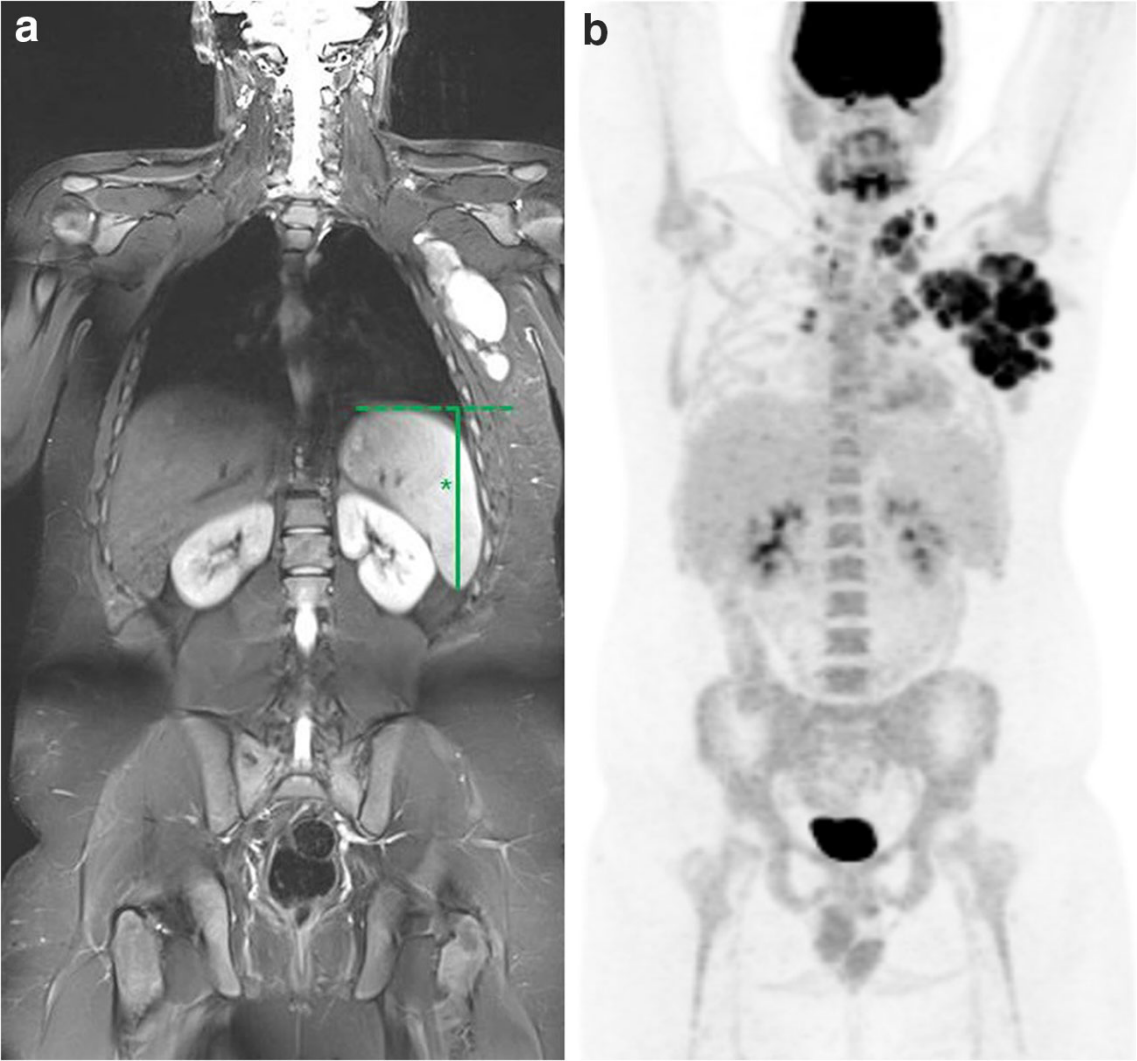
A 17-year-old boy with stage II Hodgkin lymphoma. Example of upstaging by WB-MRI due to enlargement of the spleen without focal lesions. Coronal T2-weighted WB-MRI (a) shows enlargement of the spleen without focal lesions (*size = 14.1 cm). Coronal maximum intensity projection (MIP) of the FDG-PET (b) demonstrates no involvement of the spleen. The involved lymph node stations are cervical, axillary, infraclavicular, mediastinal and hilar (b)
